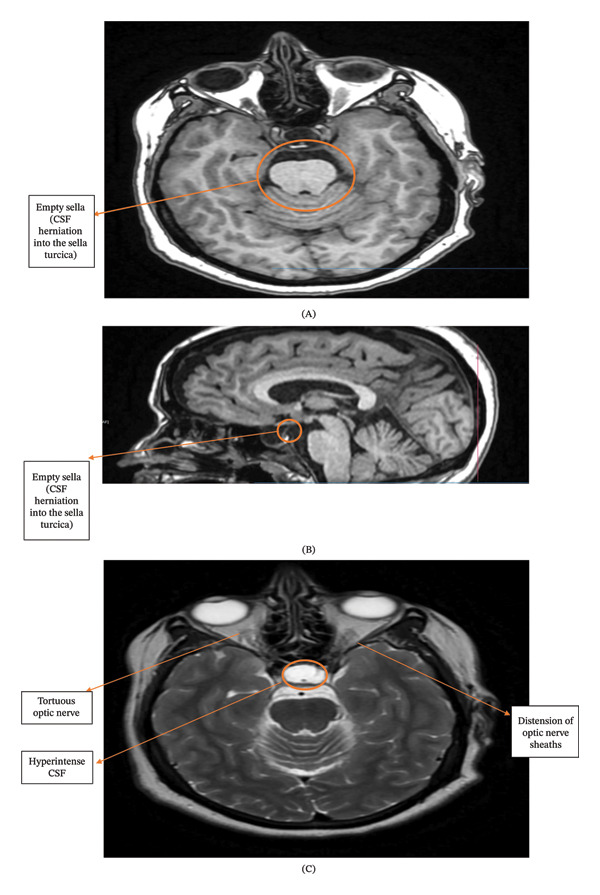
Neuroimaging findings of empty sella turcica and associated signs of idiopathic intracranial hypertension. (A) Axial T1‐weighted MRI showing cerebrospinal fluid filling the sella turcica with compression and flattening of the pituitary gland along the sellar floor. (B) Sagittal T1‐weighted MRI confirming sellar enlargement and cerebrospinal fluid herniation. (C) Axial T2‐weighted MRI demonstrating hyperintense cerebrospinal fluid signal within the sella, consistent with empty sella and bilateral distension of the optic nerve sheaths with tortuous optic nerve (consistent with idiopathic intracranial hypertension).
